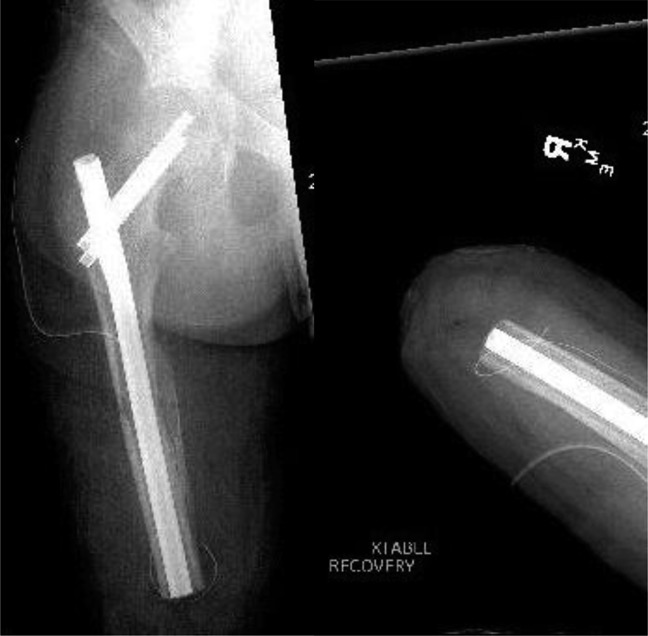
Radiographs of the right femur after above-the-knee amputation.
